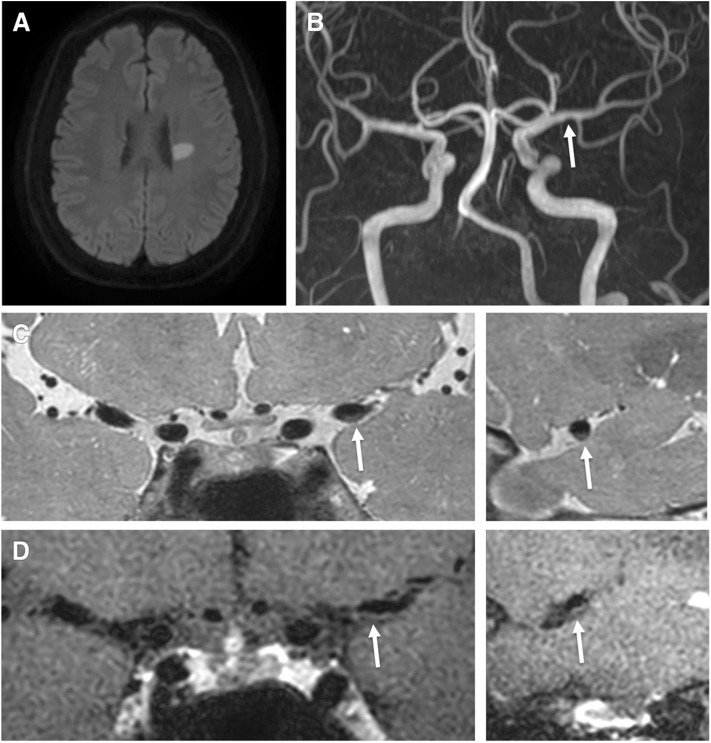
A 62-year-old male patient presented with right hemiparesis with low-density cholesterol, total cholesterol, and Apo (b) levels of 67 mmol/L, 113 mmol/L, 75.5 g/L, respectively. (A) Axial DWI shows a high signal intensity lesion in the left corona radiata region. (B) TOF-MRA shows mild stenosis in the left middle cerebral artery (proximal M1, arrow). (C) Pre-contrast 3-dimensional proton-density black-blood magnetic resonance images. (D) The post-contrast images (left: coronal plane, right: sagittal plane) show eccentric wall thickening (arrow) without enhancement.
